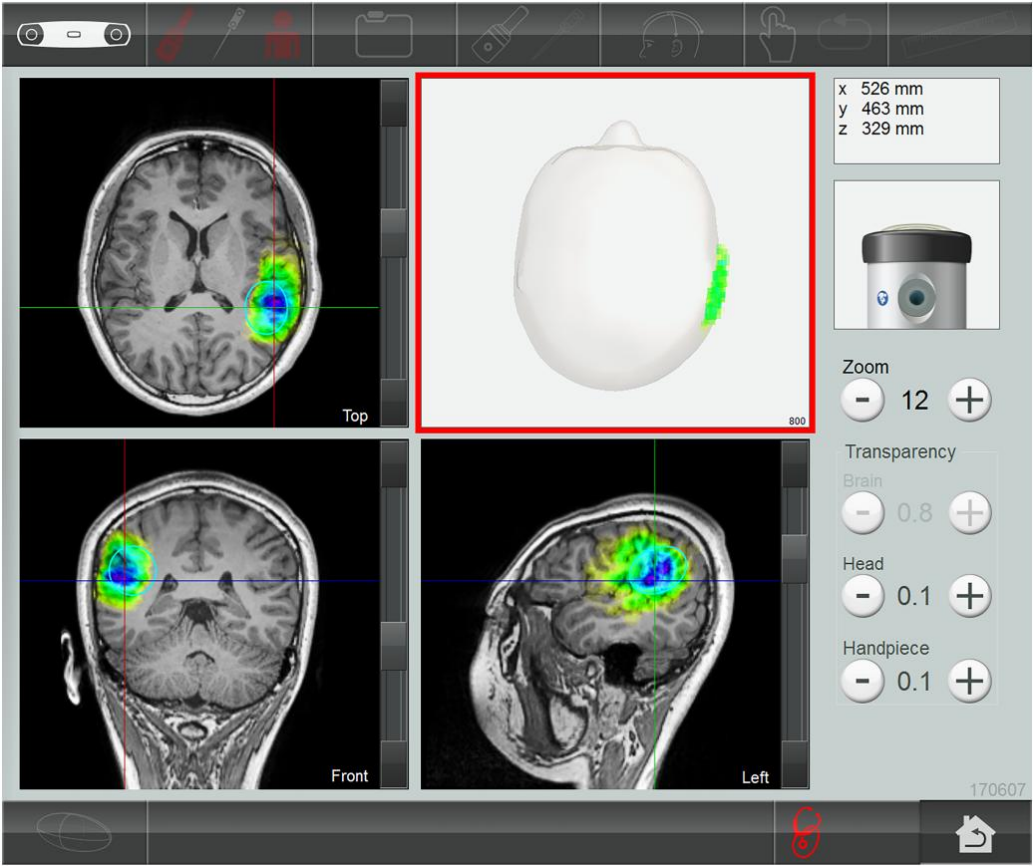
Participant’s MRI (T_1_-weighted) images. The stimulated treatment region (rTPJ) after TPS intervention.
